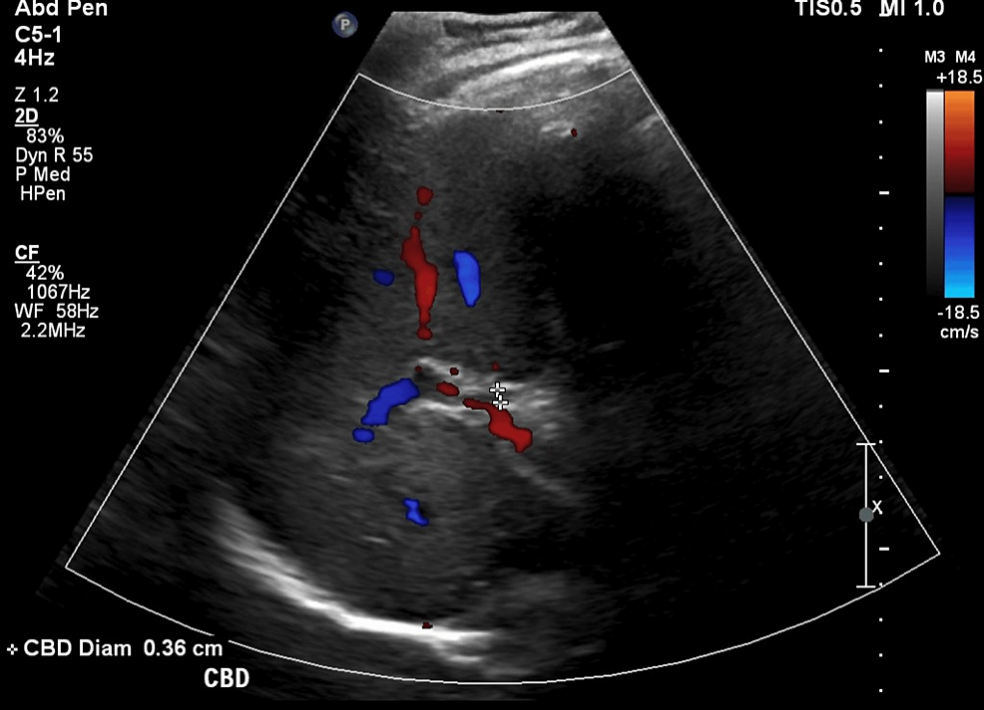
Ultrasound Abdomen Right Upper Quadrant - Common Bile Duct
